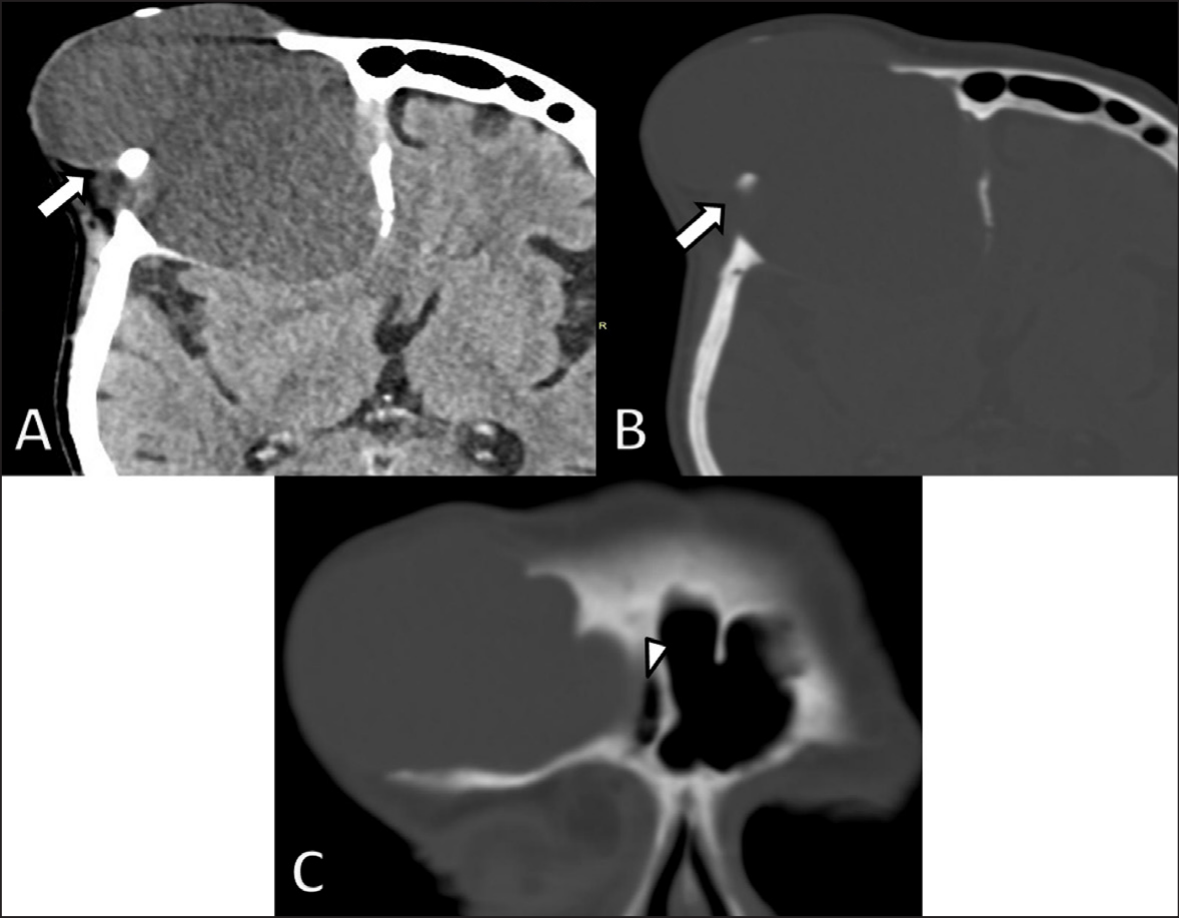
Axial non-enhanced CT images four years later. A. Soft tissue and B. bone window demonstrate considerable growth of the lesion (arrows) and a decrease in density. There is increased osteolytic destruction of the frontal bone and progressive extra-axial extension into the brain with mass effect on the frontal horn of the lateral ventricle. C. Coronal reformatted CT image shows an intimate relationship of the lesion with the right frontal sinus (arrowhead).
